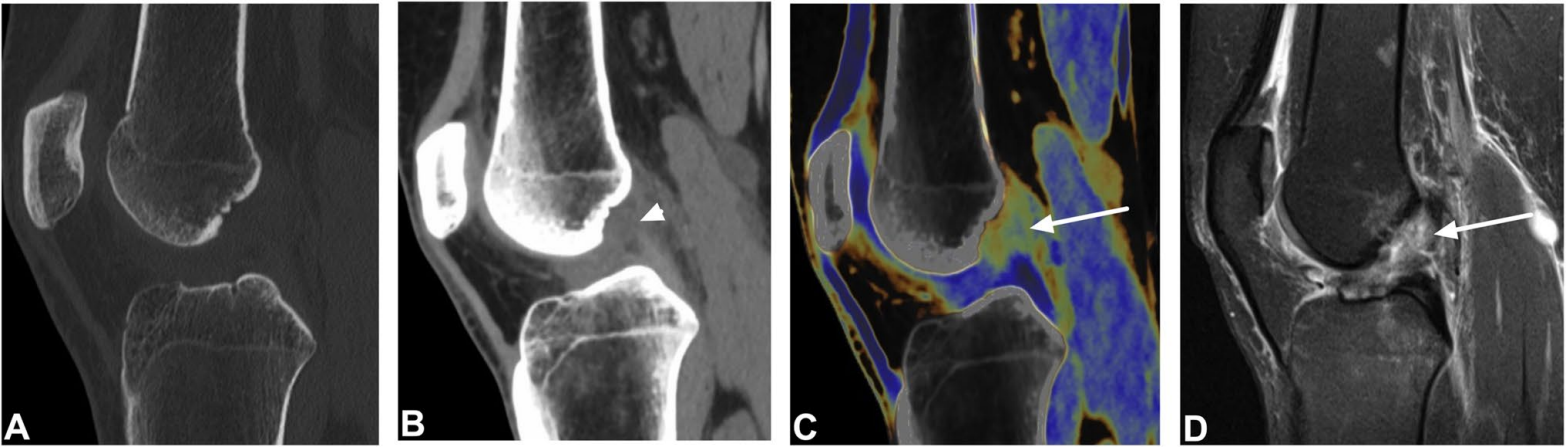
Standard sagittal unenhanced grayscale CT (A, B), color-coded collagen reconstructions (C), and proton density-weighted unenhanced MRI series with fat saturation (D) of a complete tear (arrows) of the anterior cruciate ligament (ACL) in the cranial part in a 31-year-old professional kickboxer who repeatedly twisted her knee during kickboxing and presented to the emergency department with knee pain on Friday night immediately after training. Standard grayscale CT images showed no presence of fracture. However, a subtle inhomogeneity of the ACL in the cranial aspect (arrowhead) was noted. Color-coded collagen maps showed a distinct lack of collagen signal in this area (arrow) indicating a complete tear of the ACL. Subsequently, the additionally performed MRI confirmed the diagnosis, which was treated the next morning by arthroscopic repair. Abbreviations: ACL, anterior cruciate ligament
